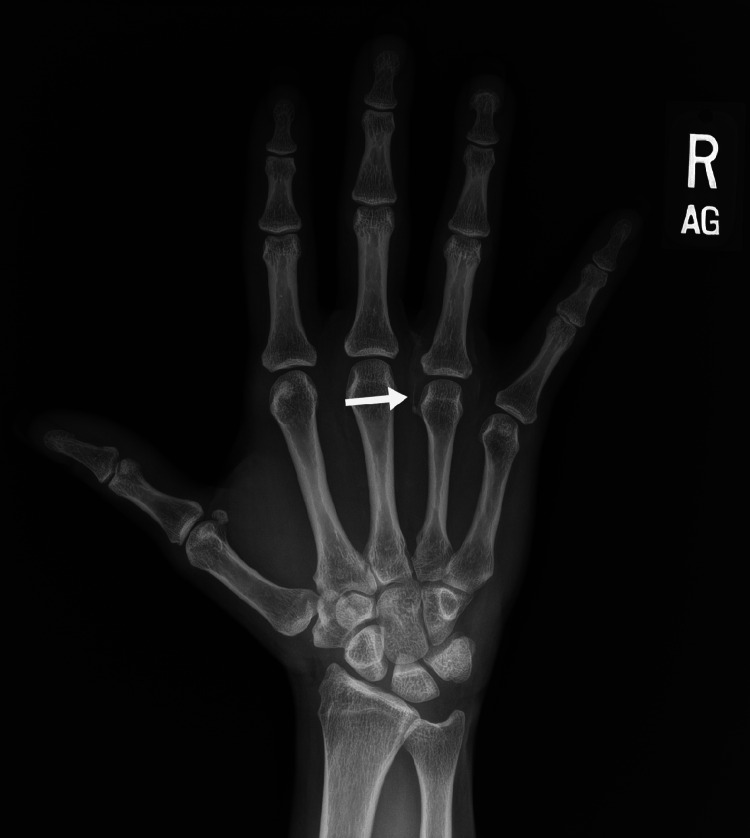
Frontal radiograph of the right hand demonstrates minor residual calcification around the ring finger MCP joint (arrow), markedly improved when compared with Fig. 1.
